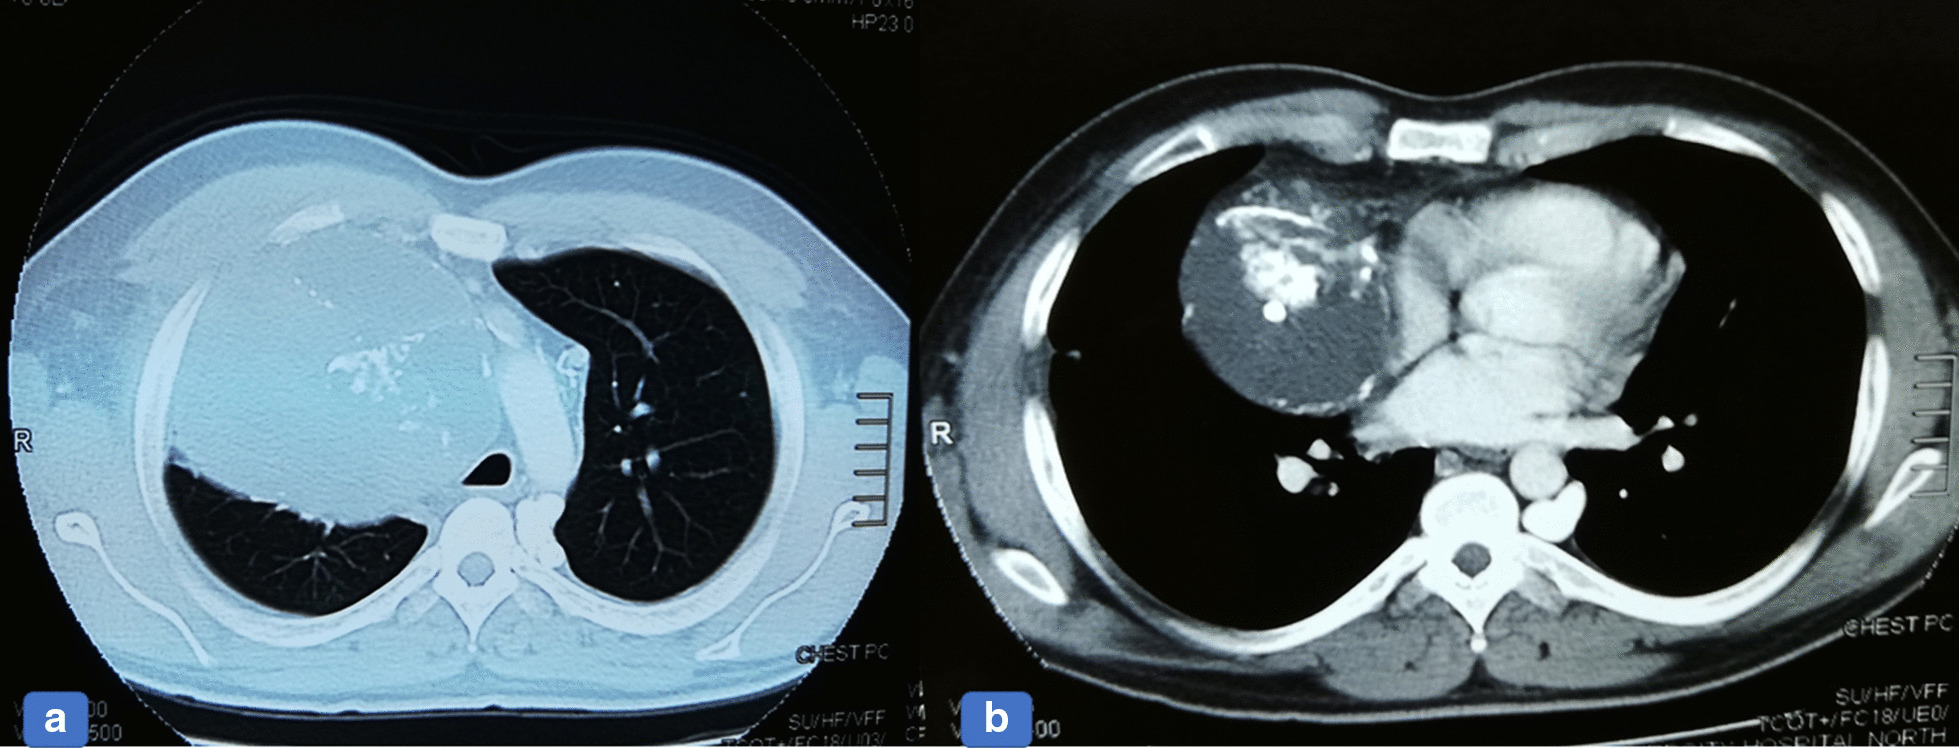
a, b Axial images of the chest-lung computed tomography and mediastinal window show well-defined rounded low attenuation mass with internal coarse calcification.
